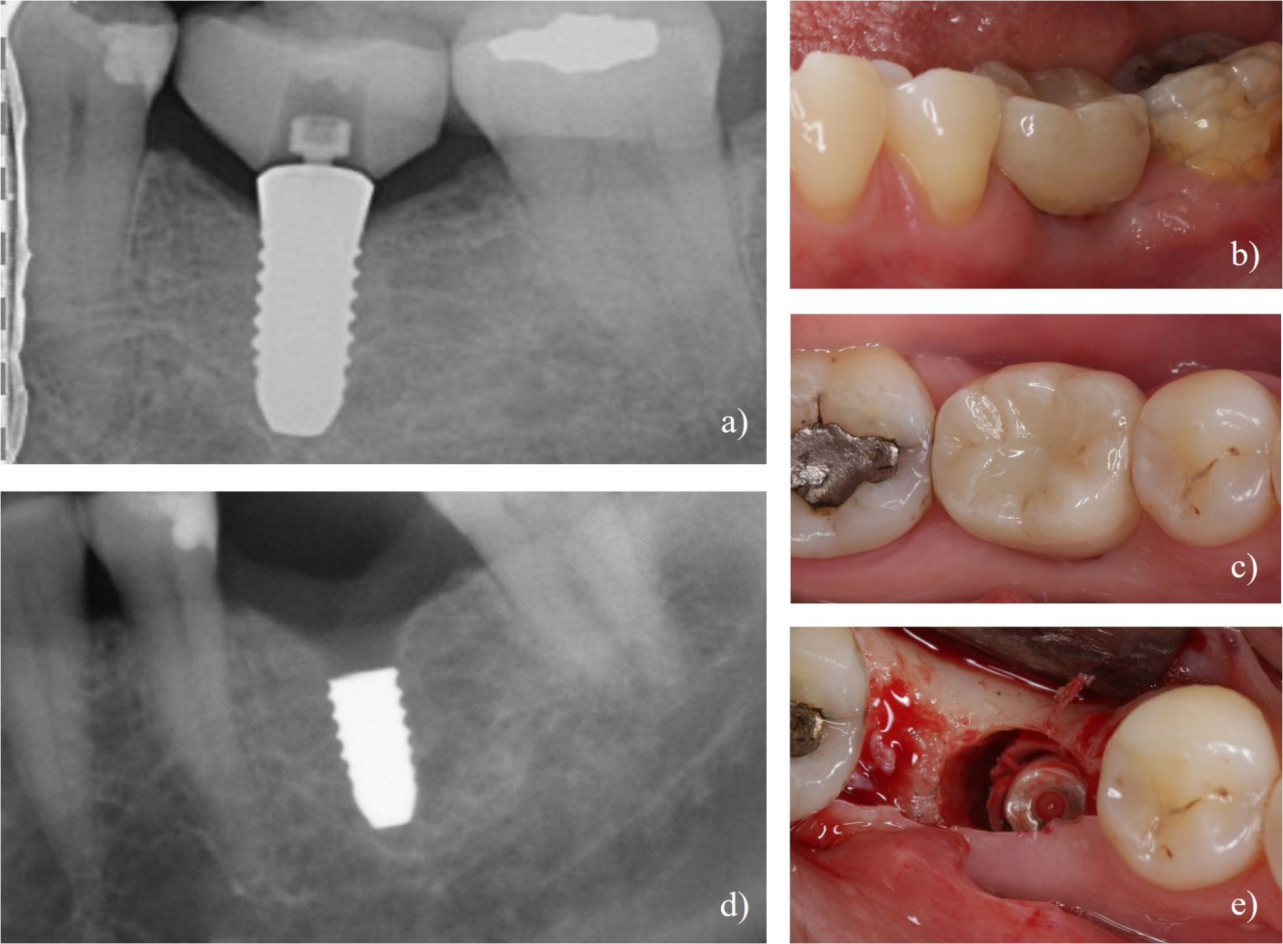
Case of implant fracture. a Initial radiograph showing dental implant with definitive screw retained crown and translucent PEKK abutment. b, c Clinical view of implant-supported crown in situ at time of definitive prosthesis placement. d Follow-up radiograph revealing fracture of coronal third of implant. e Clinical appearance after implant fracture, showing the fractured implant in situ
